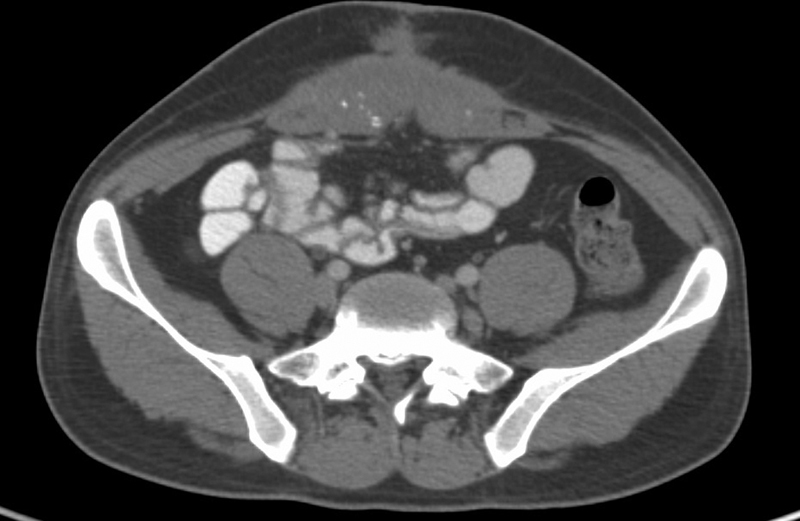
Contrast enhanced CT scan Abdomen showing umbilical lesion.
